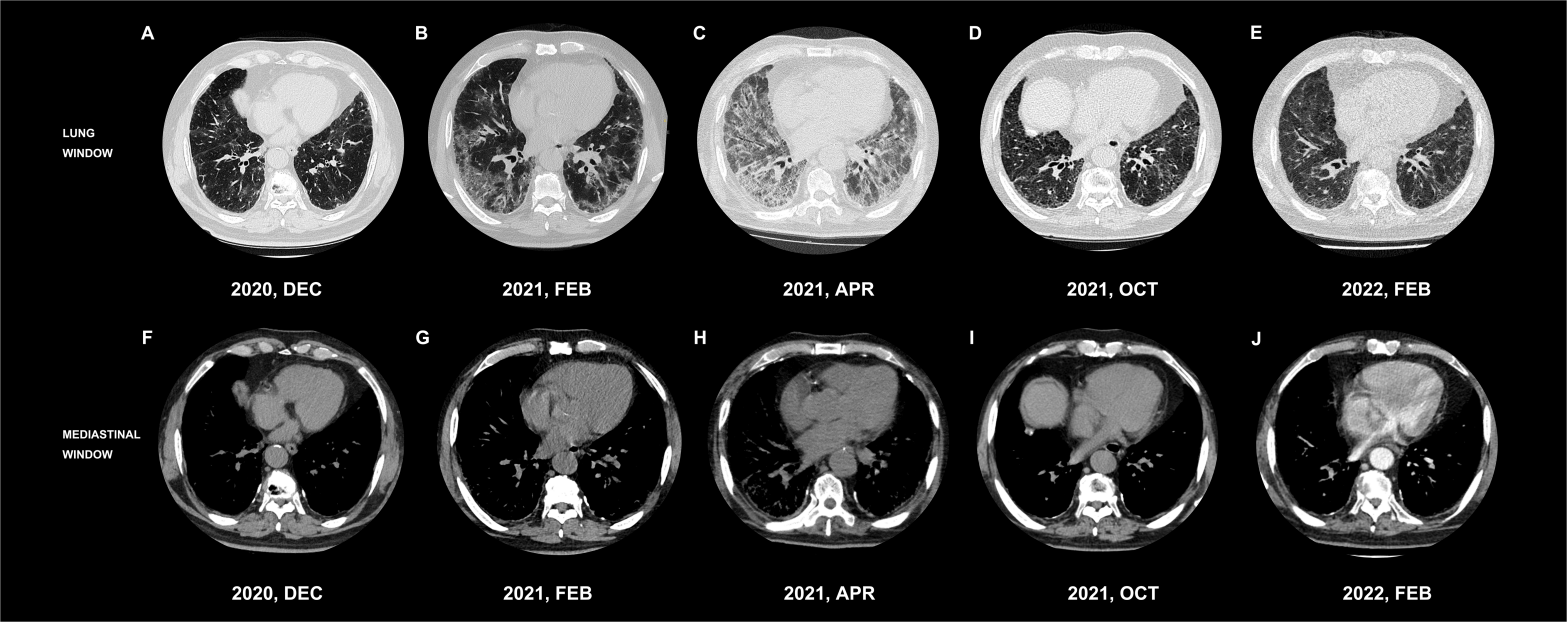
Chest CT of inferior lungs in the lung and mediastinum windows. Dynamic changes in the interstitial lesions in the bilateral inferior lobes of the lung. (A)/(F) Mild basic interstitial changes. (B)/(G) Significant peribronchovascular and subpleural GGOs, reticulation, and consolidation. (C)/(H) Significant fibrotic with GGOs, reticulation, consolidation, honeycomb shadows, and thickened interlobular septa. (D)/(I) Significantly improved interstitial changes and better transparency. (E)/(J) Stable mild interstitial changes.
